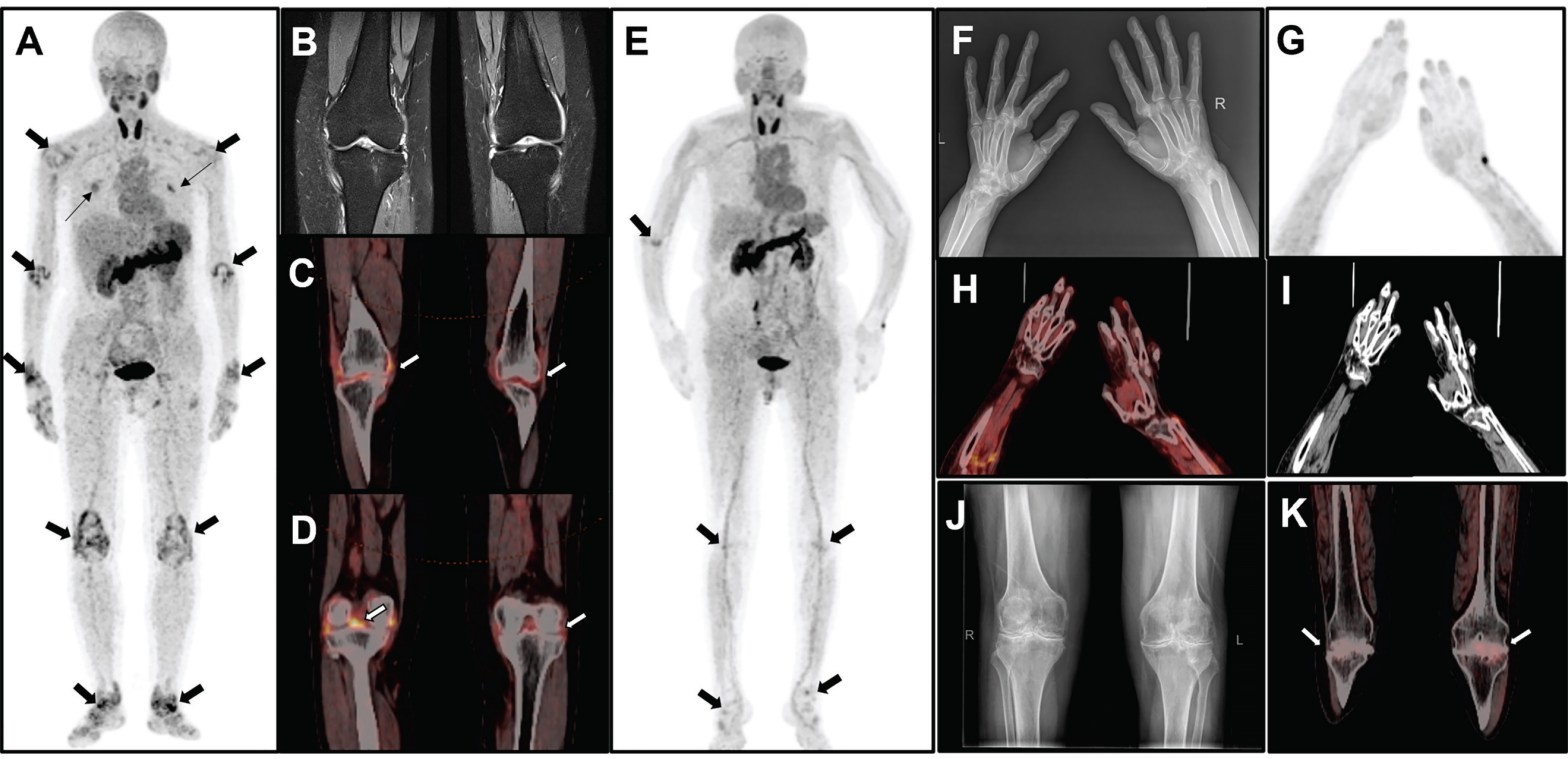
The MIP image (A) obtained from a 51-year-old female participant (No. 2) displays increased uptake in multiple joints (thick arrows) and muscles (thin arrows). The participant has DAS28-ESR score of 6.9, DAS28-CRP score of 5.9, CDAI of 38.0, and SDAI of 39.4. Bilateral knee MRI T2 images (B) reveals thickening of the knee capsule, suprapatellar bursa, and popliteal synovium. Axial PET/CT fusion images (C and D) demonstrate increased uptake of FAPI-RGD in these specific areas. The MIP image (E) of a 69-year-old female participant (No. 18) reveals mild to moderate increased uptake in various joints (thick arrows). The participant has DAS28-ESR score of 4.4, DAS28-CRP score of 3.8, CDAI of 10.0, and SDAI of 32.1. X-ray imaging (F) of both hands and wrists reveals slightly narrowed and blurred interphalangeal joint spaces, soft tissue swelling around the joints, cystic degeneration in both hands and wrists, and an unclear wrist joint space. MIP image (G) alongside PET/CT images (H, fusion; I, CT) of the hand and wrist region reveals osteoporosis and some deformities, with no significant uptake at the corresponding sites. Bilateral knee radiograph (J) shows bilateral tibial plateaus, femoral epicondyles and patellar rim osteophytes, with significant narrowing of the joint space, local fusion, and uneven bone density under the articular surfaces. The axial PET/CT fusion image (K) shows a bilateral narrowing of the knee joint space and an increased mild FAPI-RGD uptake.
